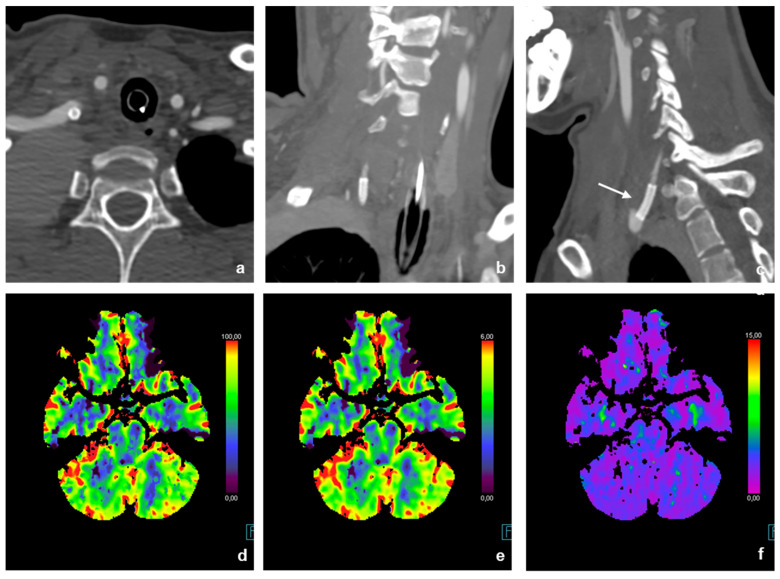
Postinterventional CT-A and CT-P. Correctly inserted BeGraft Stent in the V0-Segment of the right vertebral artery ((a–c), indicated by a white arrow). The postinterventional cerebral CT–P controls showed no focal ischemia (d–f).
